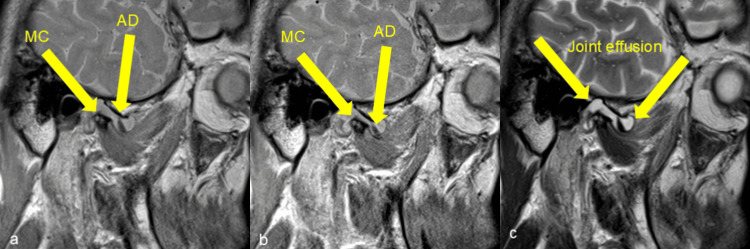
Magnetic resonance images of the right temporomandibular joint. (a) Sagittal proton density image during mouth closing. Anterior disc displacement was observed. (b) Sagittal proton density image during mouth opening. Anterior disc displacement without reduction was observed. (c) Sagittal T2-weighted image during mouth closing. A well-defined hyperintense region showing joint effusion was observed. MC: mandibular condyle; AD: articular disc
